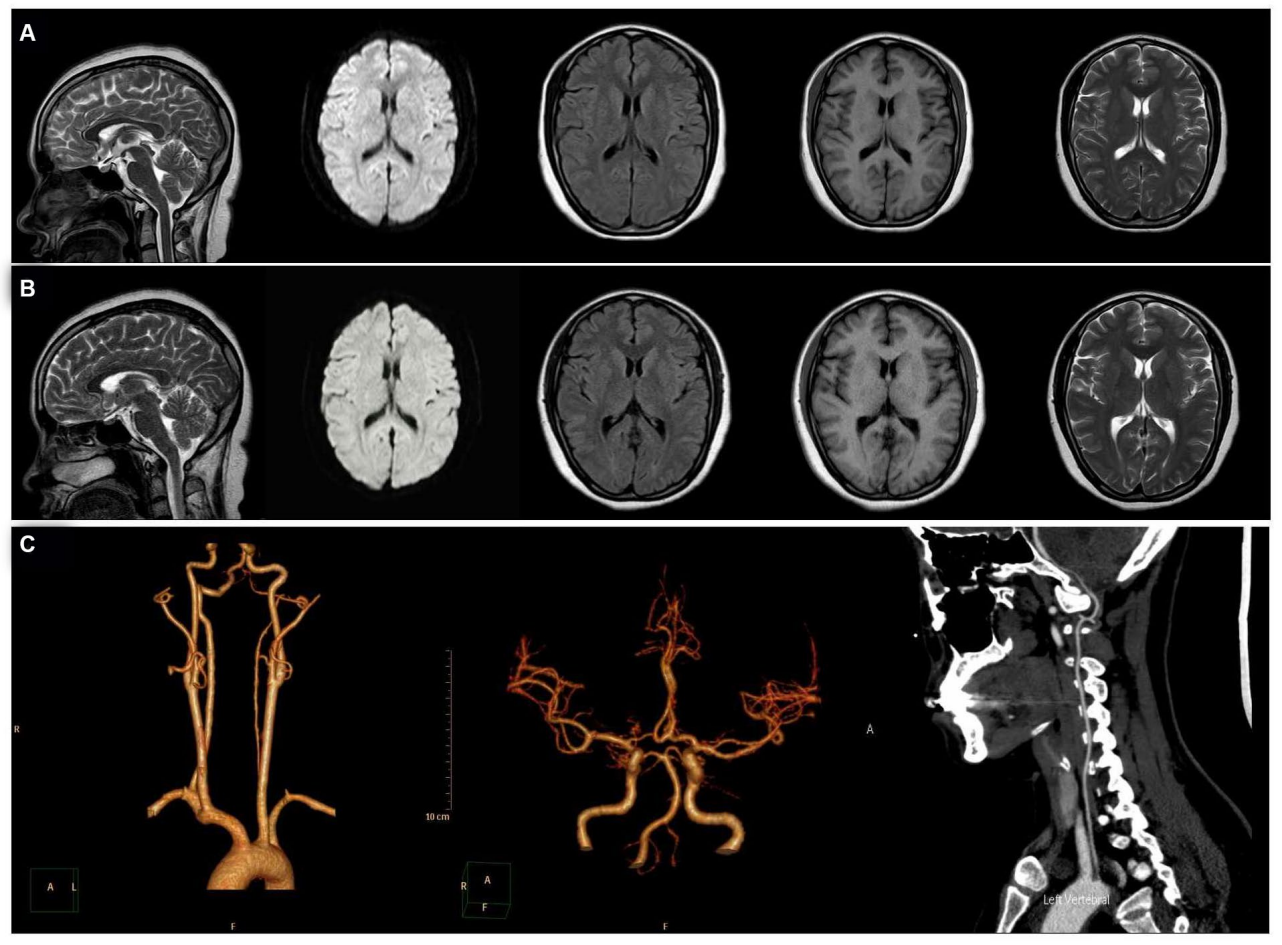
Magnetic resonance imaging (MRI). From left to right: sagittal, diffusion-weighted, fluid-attenuated inversion recovery, T1, and T2 sequences. (A) MRI showed no significant abnormalities during headache attacks with hemiparesis. (B) MRI showed no significant abnormalities at 6 months after patent foramen ovale closure. (C) Computed tomography angiography revealed left vertebral artery hypoplasia.
